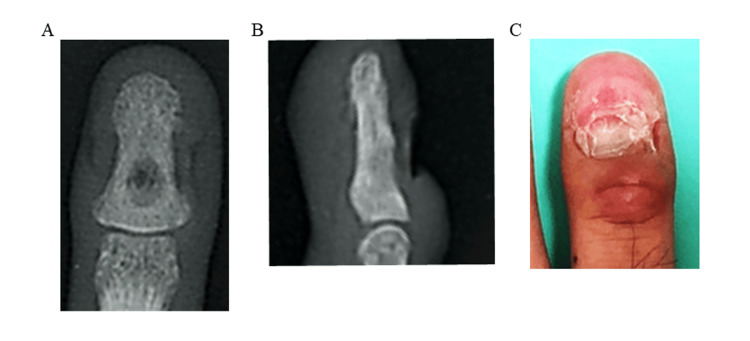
Postoperative radiographs and gross appearance of the distal right ring finger at two months A: Anteroposterior view showing no evidence of tumor recurrence and partial bone regeneration; B: Sagittal view confirming no recurrence and partial bone healing;
